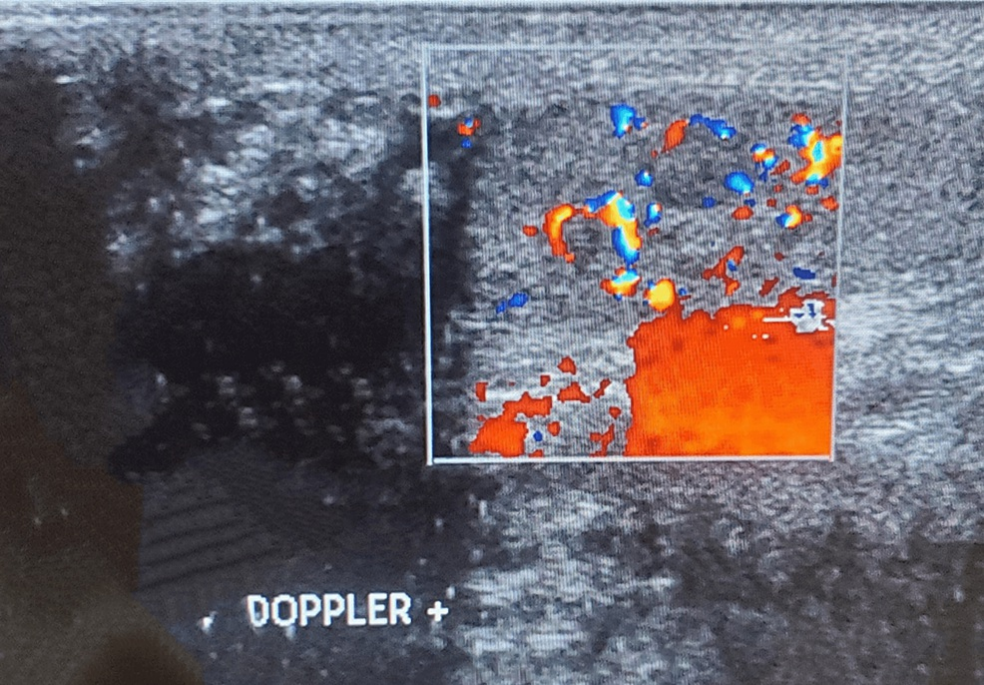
Intratesticular nodules with Doppler
